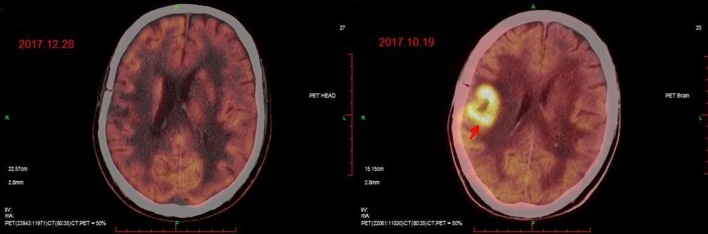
PET/CT images before (Right) and after (Left) CAR T-cell infusion. The position indicated by the arrow is the location of the lesion.
